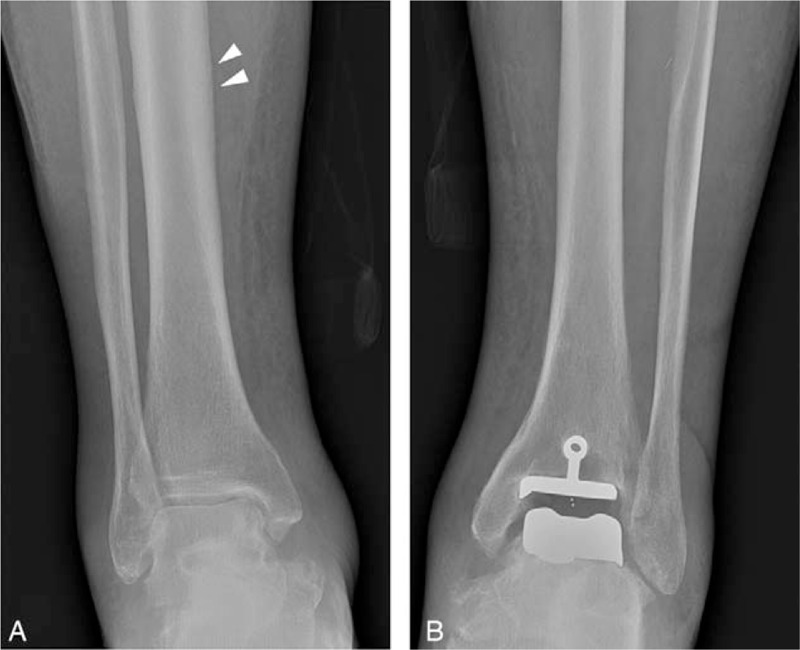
Frontal radiographs of the right (A) and left (B) lower leg 5 months earlier. There is a single lamellar periosteal reaction at the medial border of the right tibial shaft (arrowheads), which is thinner than the plain radiography in Figure 1. No bony abnormalities, except post-operative changes of the left ankle, were noted in the left lower leg. Note soft tissue swelling in both lower legs.
